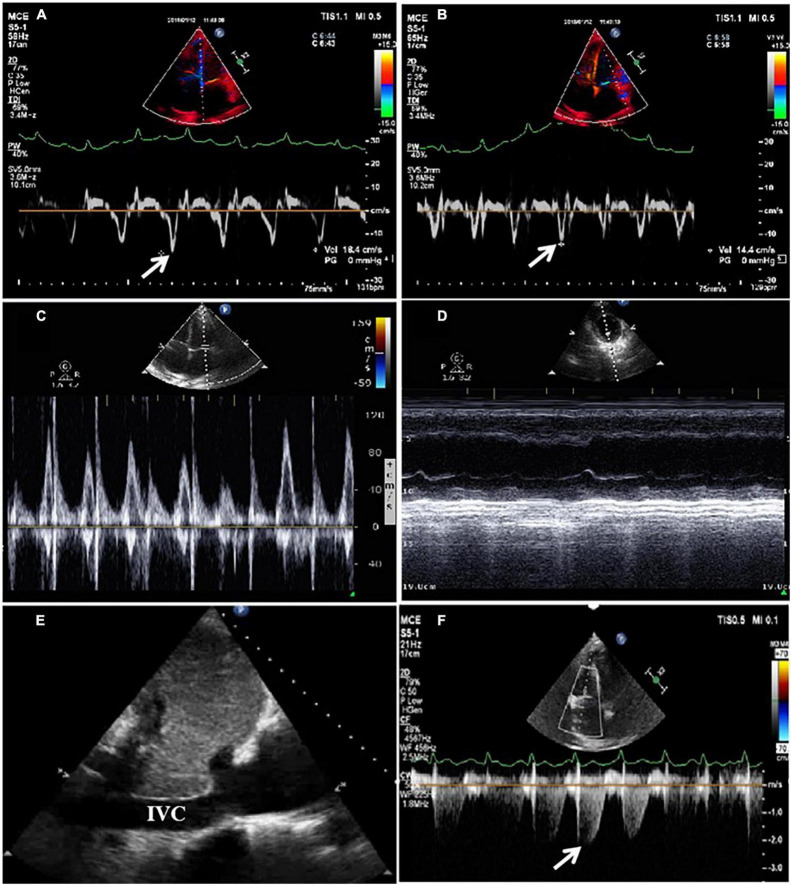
Tissue Doppler imaging (TDI) using echocardiography revealed that e’ = 18.4 m/s (medial) > 14.4 m/s (lateral) in the mitral valve (A,B). Significant respiratory variation of mitral or tricuspid peak E velocity was indeterminable due to atrial fibrillation (AF) (C). Paradoxical septal motion during respiration was not observed (D). The inferior vena cava (IVC) was dilated without inspiratory collapse (E). Pulmonary artery pressure was elevated according to the velocity of tricuspid regurgitation (F).
